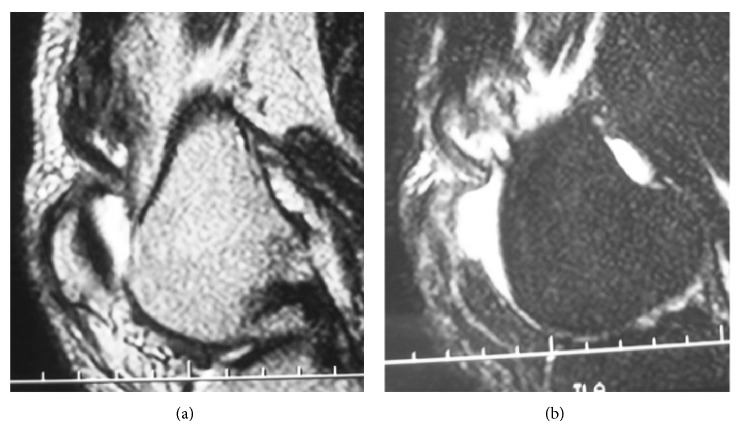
MRI findings. (a) A proton density-weighted image. Continuity of the quadriceps tendon to the patella was completely disrupted. (b) A T2-weighed fat-suppressed image. There was a high-intensity area in the midsubstance of the quadriceps tendon.
